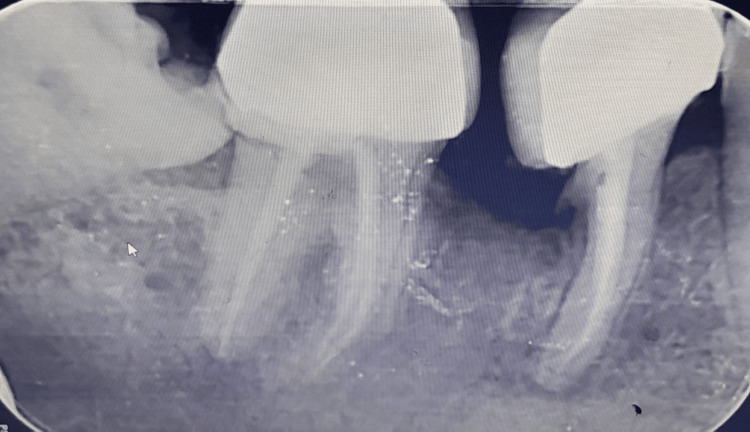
Follow-up radiograph after six months
